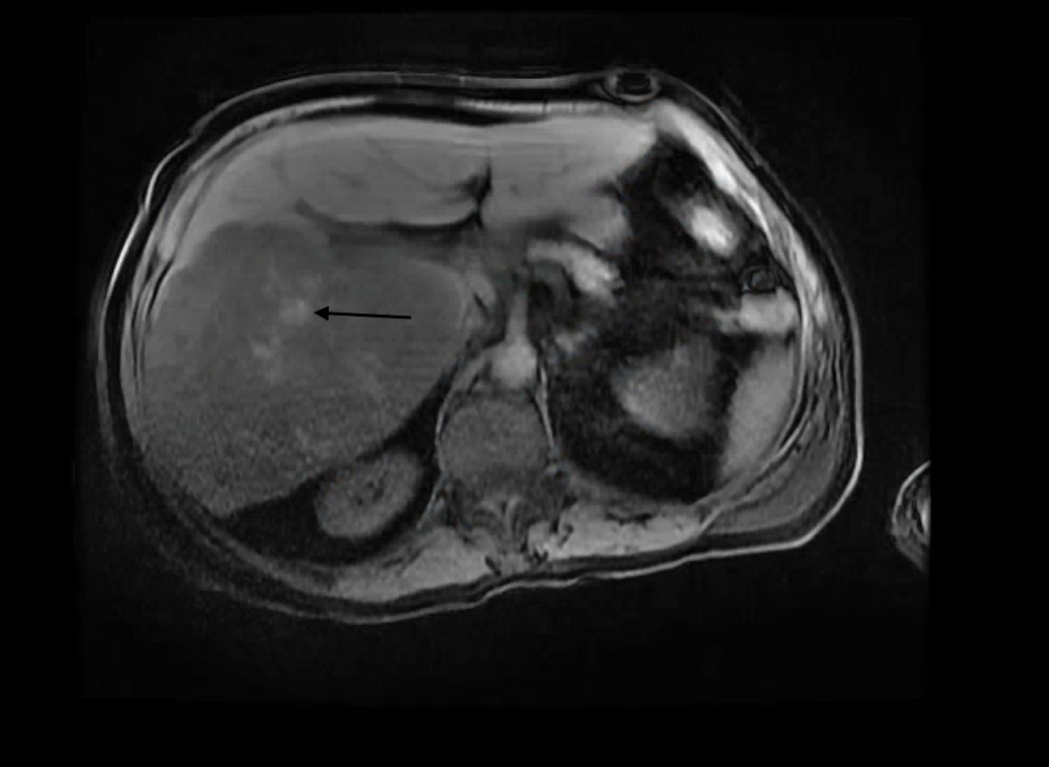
T1 Lava Flex Breath Hold Magnetic Resonance Imaging (Axial) T1 Lava Flex breath hold magnetic resonance imaging (MRI) of abdomen axial view demonstrates diffuse T1 hypointense signal through the right hepatic lobe with central areas of T1 hyperintensity (arrow). These findings represent central necrosis and hemorrhage.
